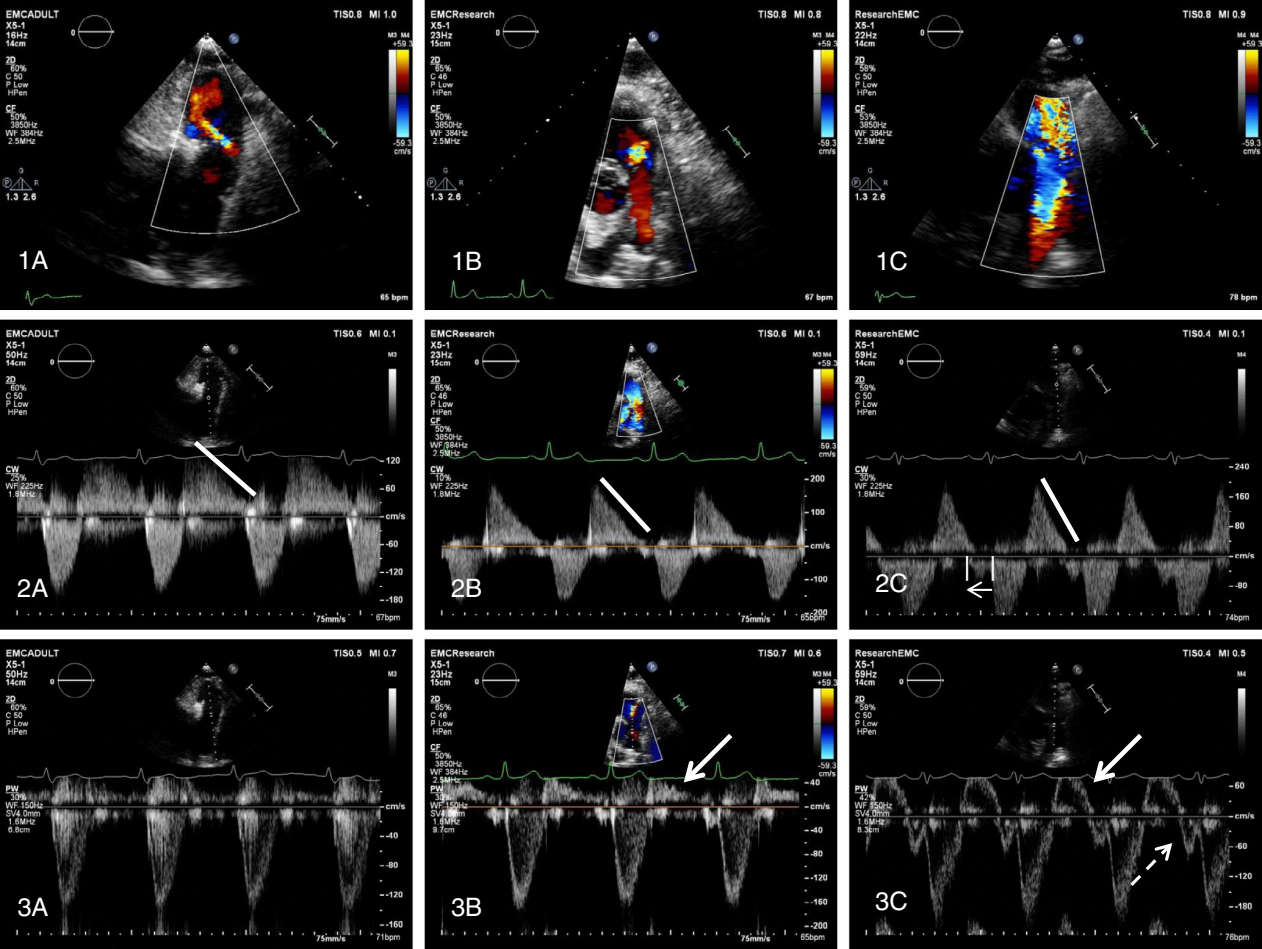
Differentiation of PR severity using color flow, CW, and PW Doppler echocardiography. Assessment of PR severity seen in the parasternal short‐axis view in a patient with mild (left), moderate (center), and severe (right) PR. Top: Qualitative assessment of PR severity using color‐flow imaging. 1A, Small regurgitant jet, no diastolic flow reversal in the main pulmonary artery (MPA) or PA branches. 1B, Increased width of the color flow jet and presence of diastolic flow reversal in the MPA. 1C, A broad color flow jet covering the total diameter of the RVOT and diastolic flow reversal coming from the right PA branch. Mid: Continuous Doppler recording of PR with PHT (white line). 2A, Slow flow deceleration of the PR jet during the entire diastole. 2B, more rapid flow deceleration during the entire diastole. 2C, rapid flow deceleration during diastole with early termination of the PR jet indicated by the small arrow. Bottom: pulsed‐wave Doppler in the MPA. 3A No signs of diastolic flow reversal in the MPA. 3B Mild diastolic flow reversal in the MPA (dense white arrow). 3C, Diastolic flow reversal in the MPA (dense white arrow) with end diastolic antegrade forward flow (dashed arrow)
